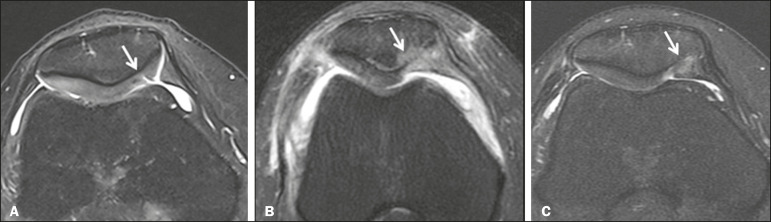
A 25-year-old woman submitted to arthroscopic injection of autologous mesenchymal stem cells into the medial aspect of the patella. Sagittal T2- weighted fat-saturated MRI sequences. Preoperative sequence (A) showing the lesion, with deep chondral fissures (arrows). Sequence obtained one week after the procedure (B), showing bone and soft-tissue edema adjacent to the repair zone (arrows), with mild joint effusion. Sequence obtained four months later (C), showing slightly irregular fibrocartilaginous tissue, with deep fissures, in the repair zone (arrows), without subchondral edema.
